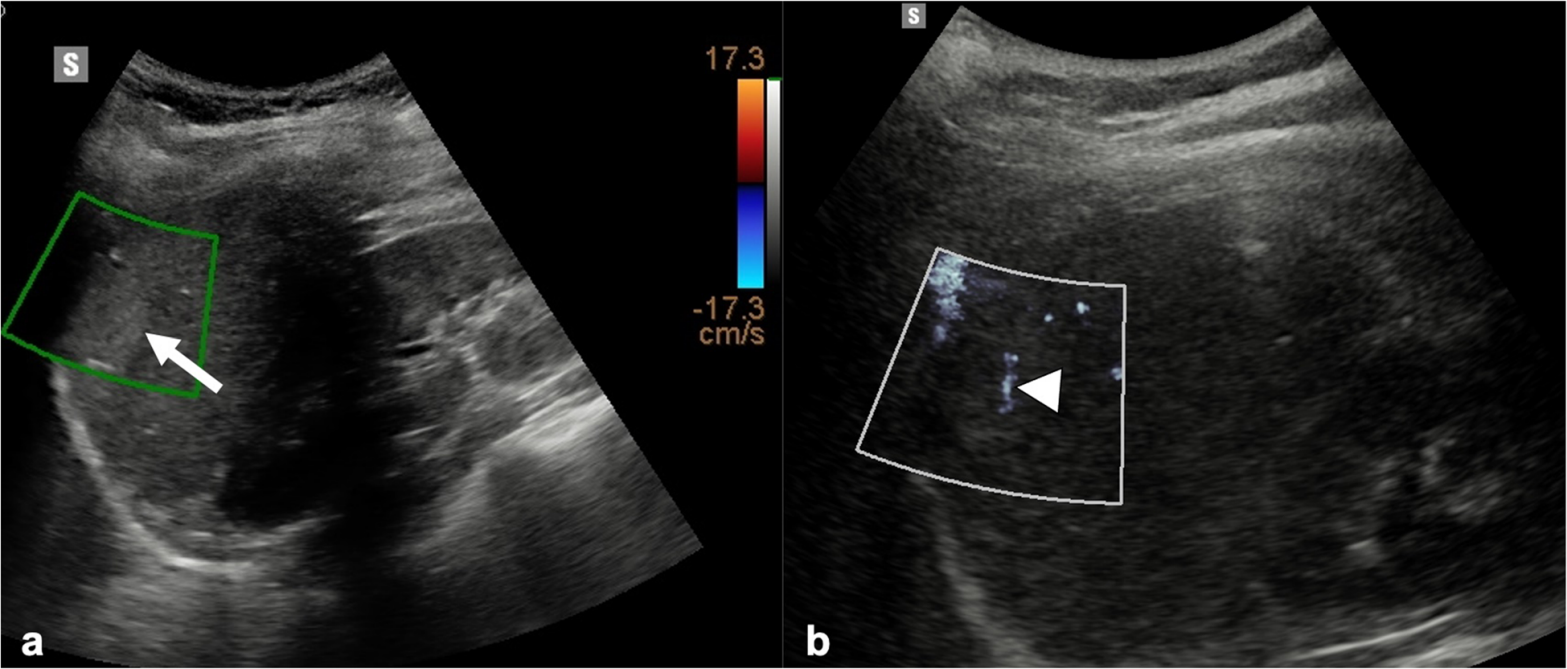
Microvascular flow imaging in a young woman with focal nodular hyperplasia. a Brightness-mode ultrasound demonstrates a 2.8-cm focal nodular hyperplasia (arrow) lacking any vascularization at conventional color Doppler. b Microvascular assessment with microvascular flow imaging clearly depicts an intralesional vessel (arrowhead)
